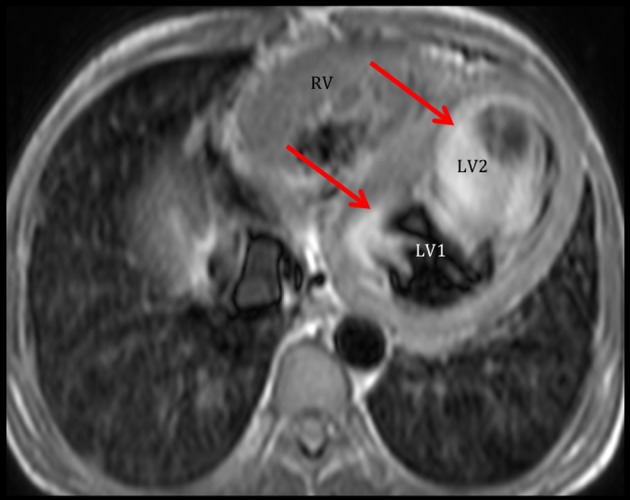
Cardiac MRI demonstrates the double chambered LV. Axial view shows that the LV is partially compartmentalized into two chambers. Note that there is marked flow stasis at the apical part of the LV (LV2) compared to that of the base (LV1). Ejection fraction by cardiac MRI was 15%.
